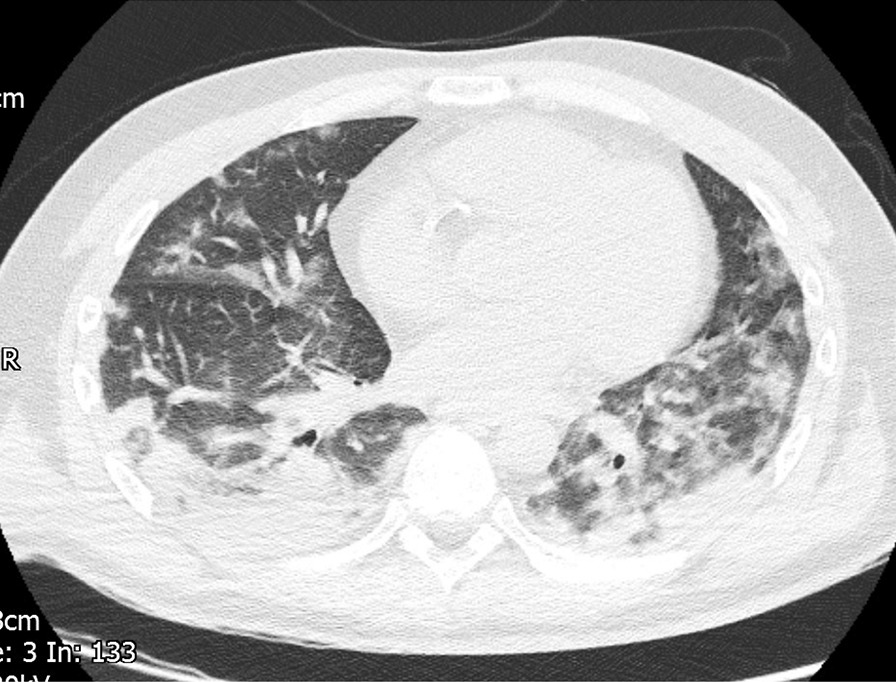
Computed tomography Thorax showing considerable covid pneumonitis
